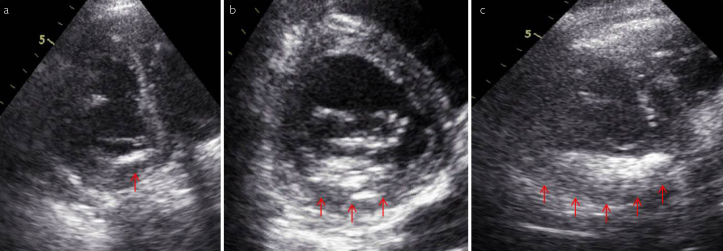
MAC positivity and echocardiographic grading. Grade 1 (mild, <3 mm thick, including less than one-third of the annulus), Grade 2 (moderate, 3–5 mm thick, less than two-thirds of the annulus), and Grade 3 (severe, >5 mm thick, intensively calcified annulus). MAC, mitral annular calcification.
